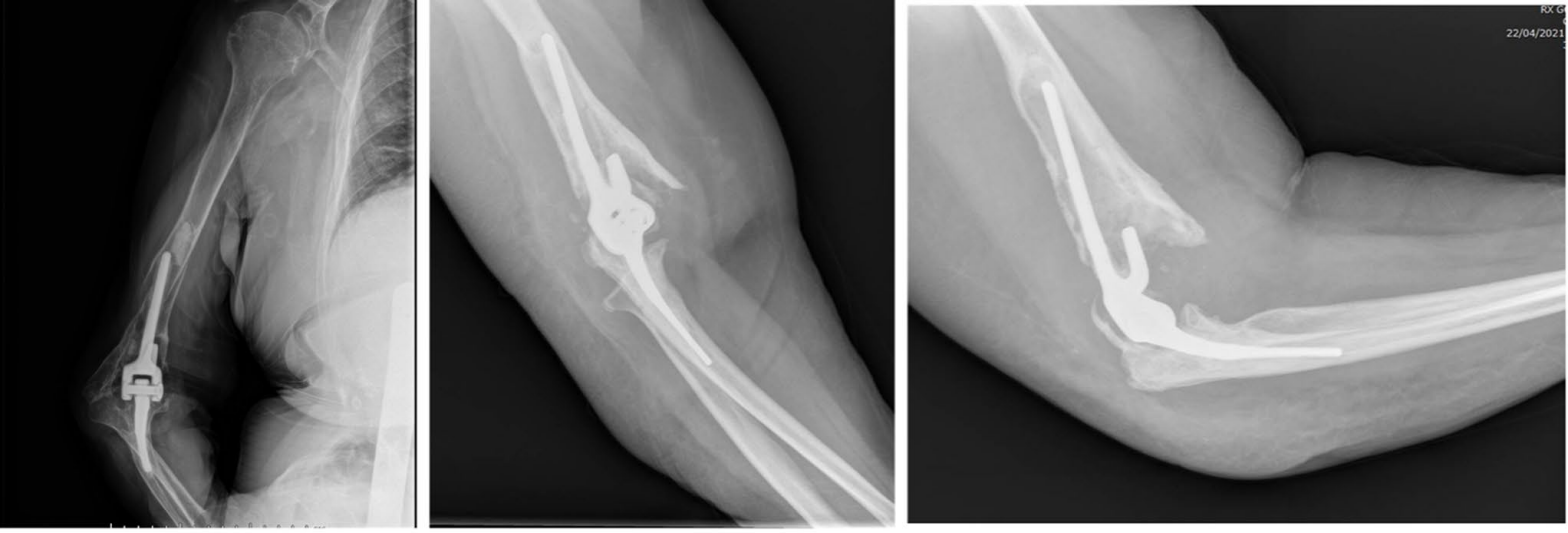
X-rays of aseptic loosening
